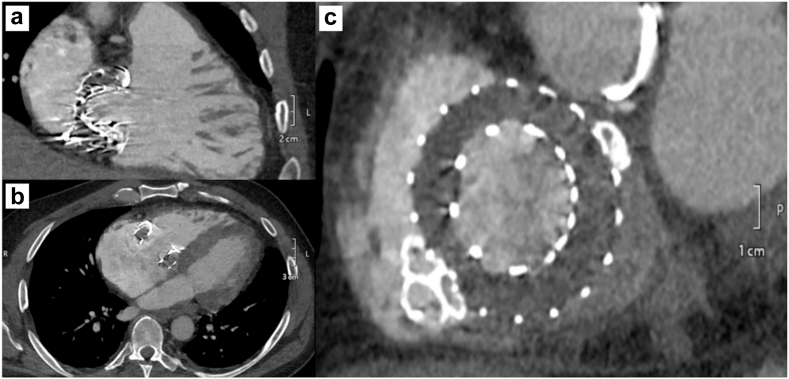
Postoperative cardiac computed tomography. (a-c) Displays the relation between the VSO devices and the EVOQUE valve. Abbreviation: VSO, ventricular septal occluder.
